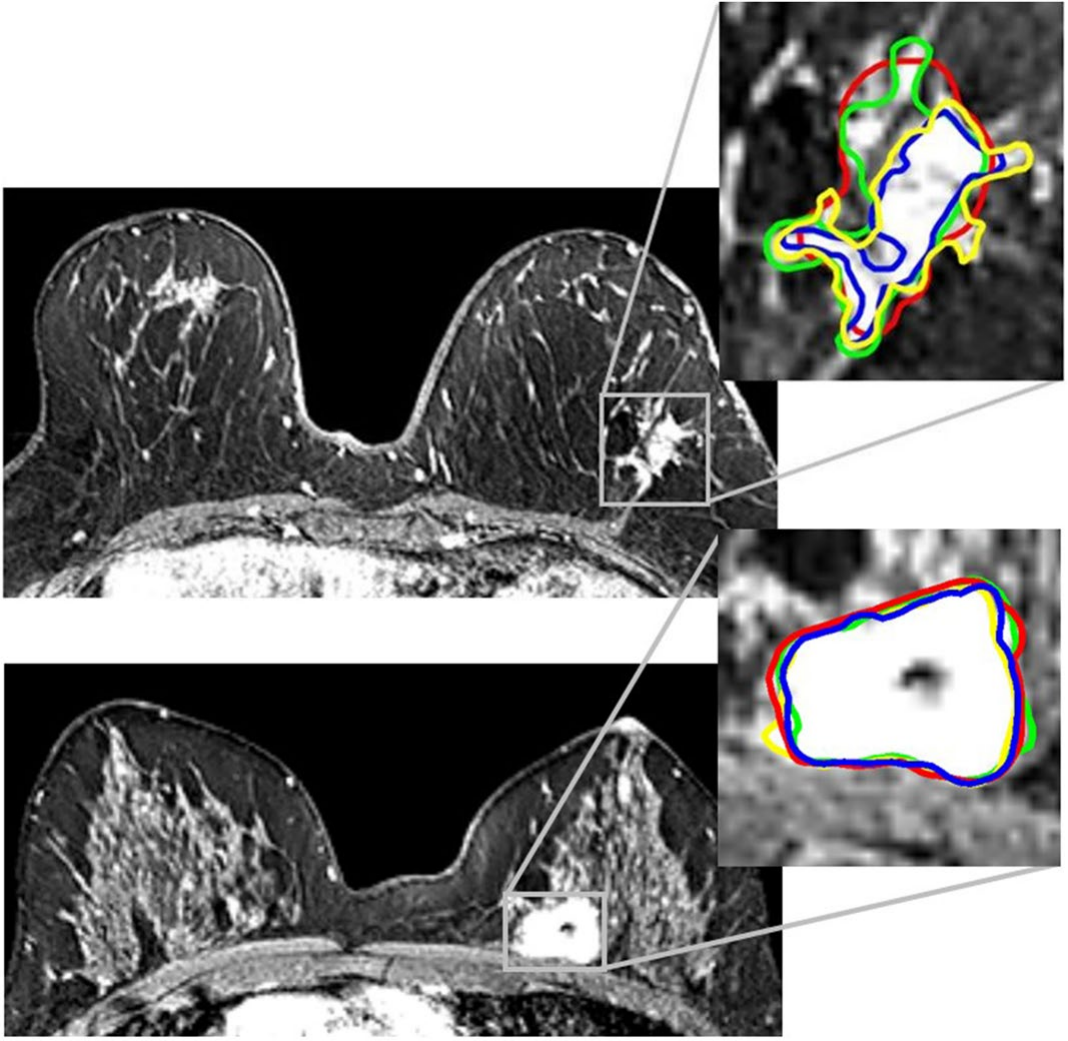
Two invasive breast tumors in the left breast on the 2-min post-contrast DCE-MRI with four single manual segmentations (colored margins: red, blue, green and yellow) fused. Upper: ‘challenging tumor’ with a mean DSC of 0.78 (range 0.71–0.82). Lower: ‘easy tumor’ with a mean DSC of 0.90 (range 0.89*–*0.91).
